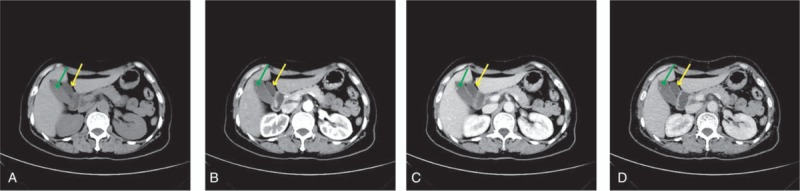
CT revealed an unusual elliptical dense mass (green arrow) with an extra tubular structure located above and medial to the native GB (yellow arrow), which measured about 47 × 21 mm^2^. CT = computed tomography, GB = gallbladder.
